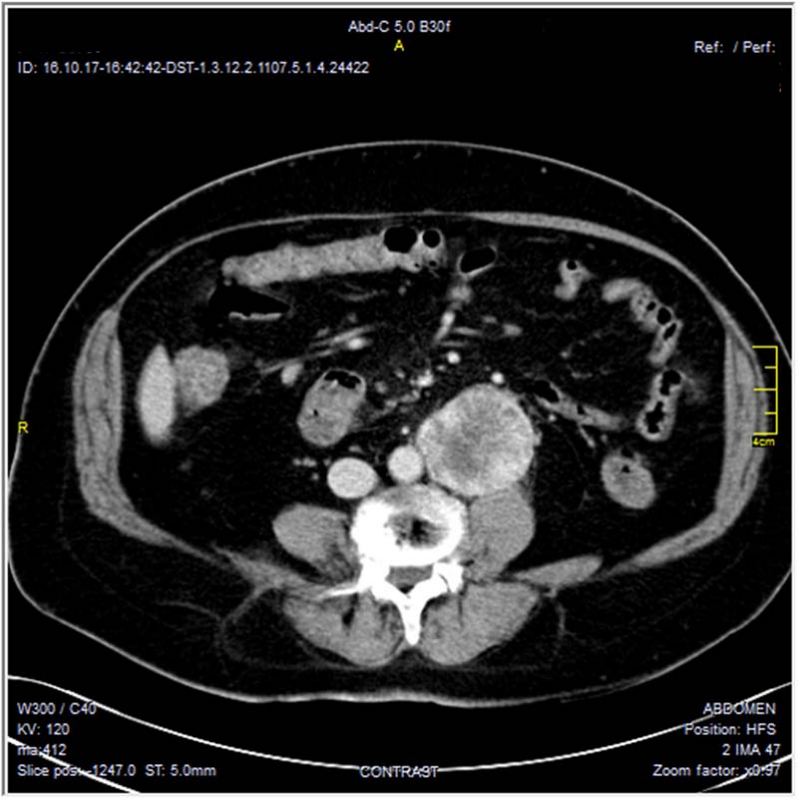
Contrast-enhanced axial CT images—soft tissue window, show well-defined peripherally enhancing mass lesion at left para-aortic region. No local invasion.
